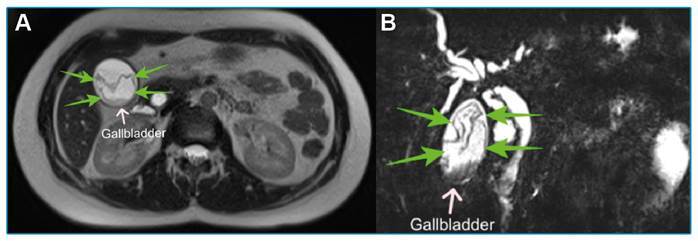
Mixed high signal membranous daughter cyst observed on T2-weighted magnetic resonance images (A) and MRCP (B) (green arrows).
